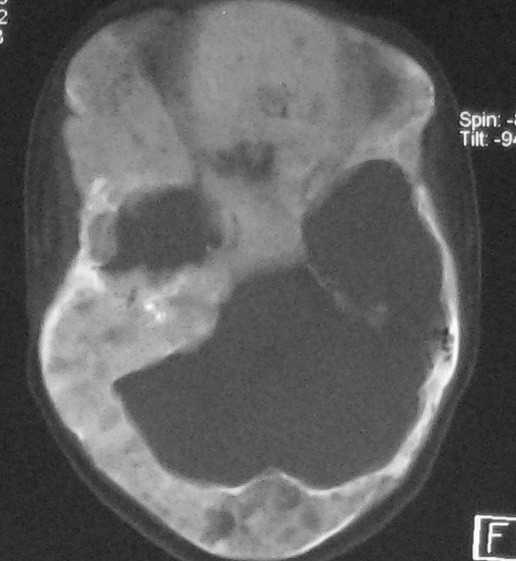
Axial CT images. There were no cyst changes in sphenoid bone 2 months ago. The density of sellar turcica is uniform.
